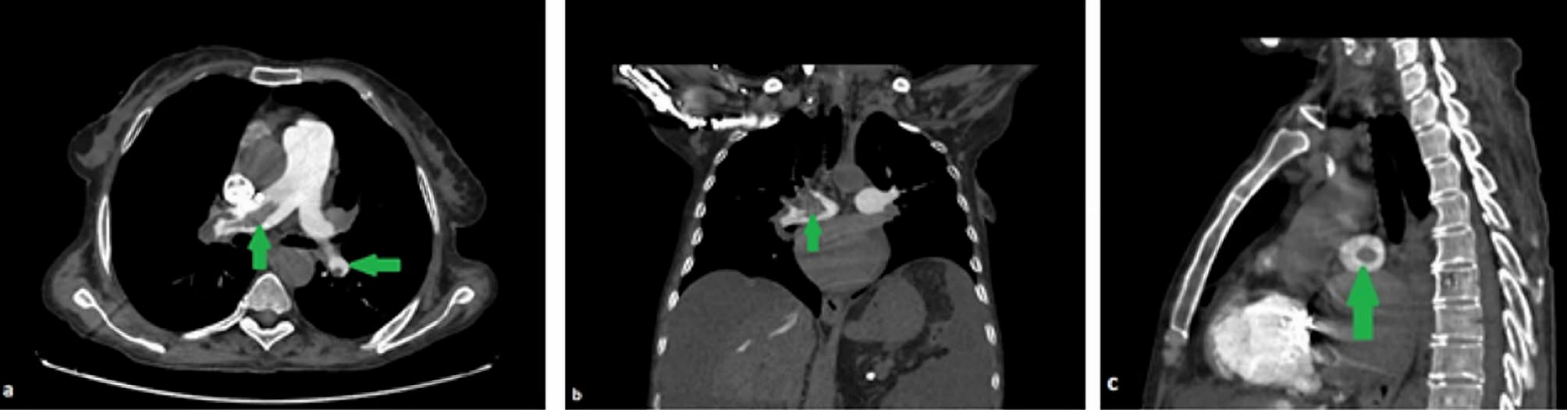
Computed tomography pulmonary angiography images of a 55-year-old female patient. Images showing a filling defect consistent with acute pulmonary thromboembolism causing < 50% stenosis in the right pulmonary artery in axial (a), coronal (b), and sagittal (c) sections (arrows)
